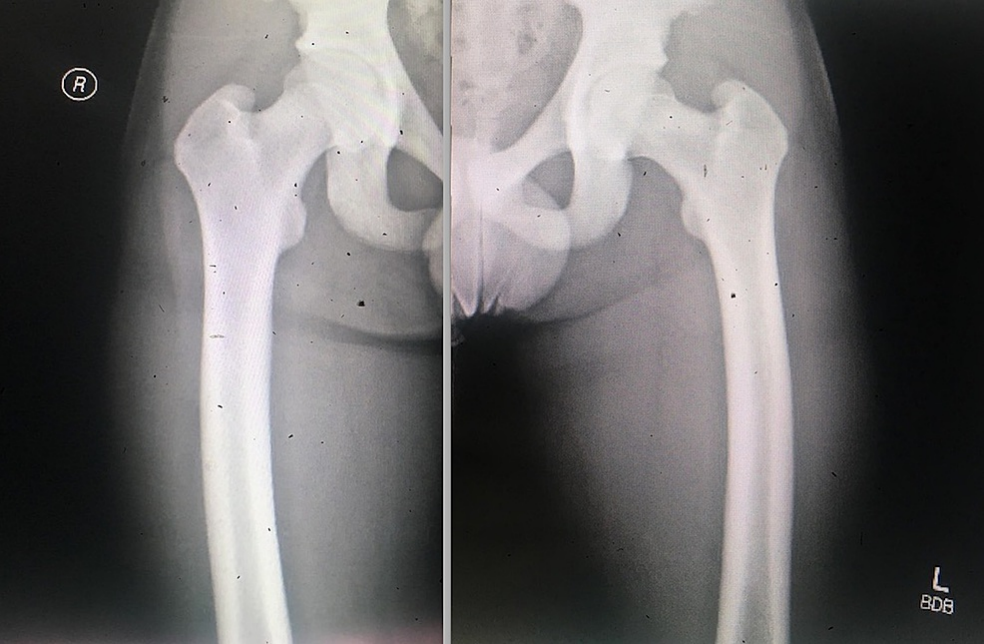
X-ray displaying symmetrical minor lateral bowing of each femoral shaft.
